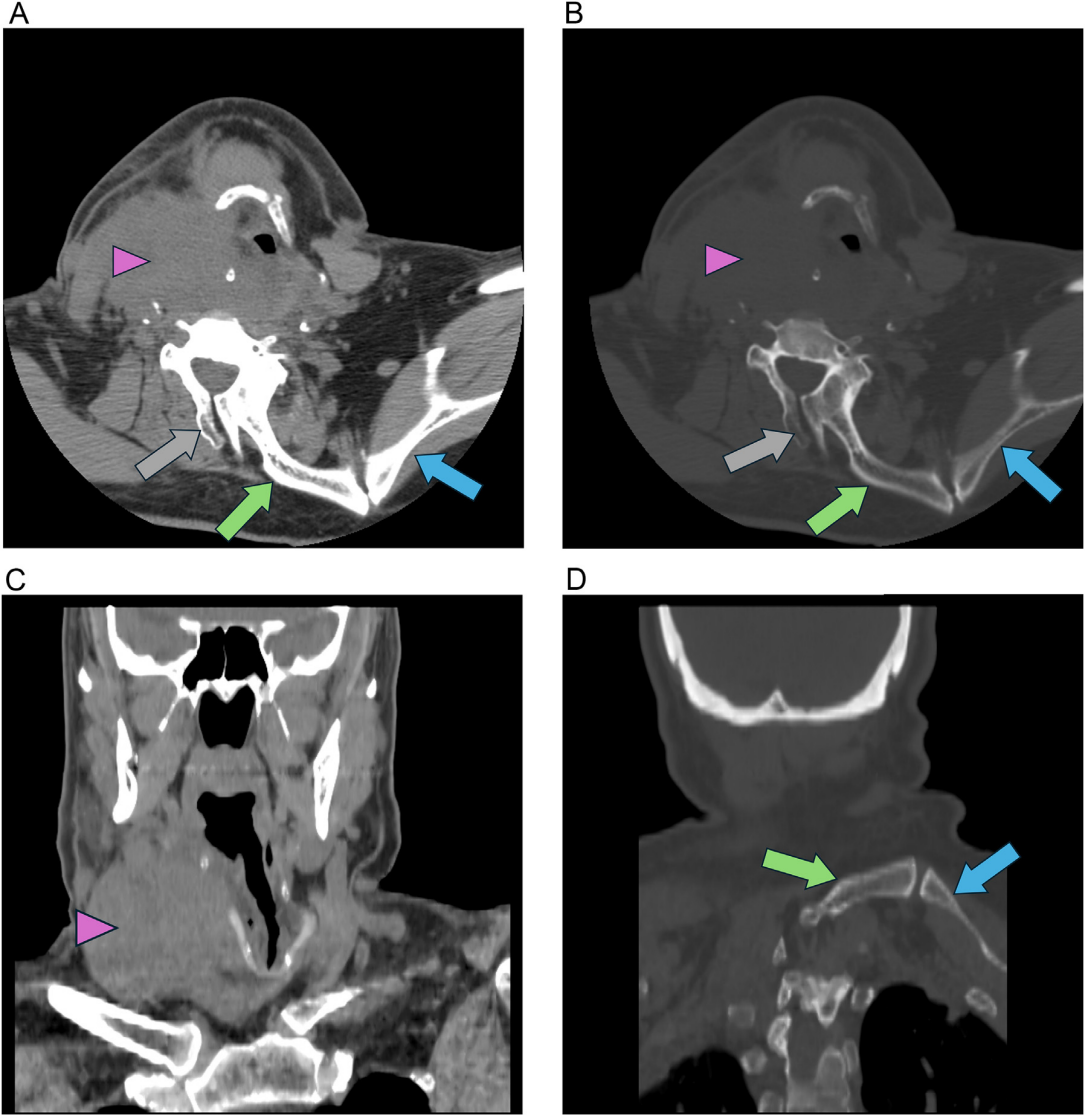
(A-D) CT of the neck shows a right neck mass (pink arrowhead) with leftward tracheal deviation. There is an abnormal bony structure extending from the C6 posterior elements to the left scapula with pseudo-articulation, consistent with an omovertebral bone (green arrow) and associated Sprengel deformity (blue arrow). An unfused cervical spinous process is seen (grey arrow). No IV contrast was administered due to renal disease.
